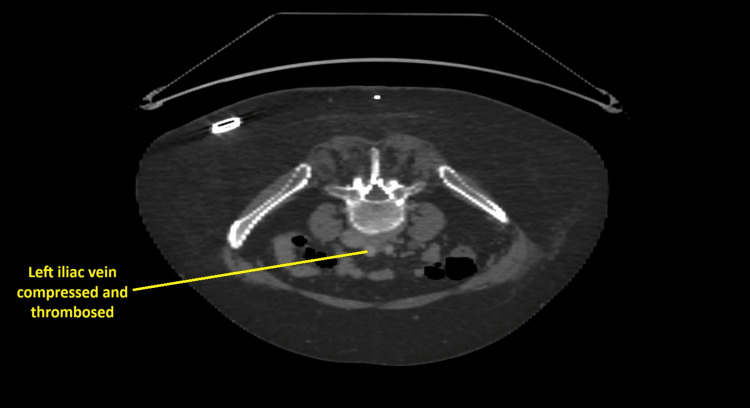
CT with contrast of the abdomen at the transverse section showing the left iliac vein compressed and thrombosed
